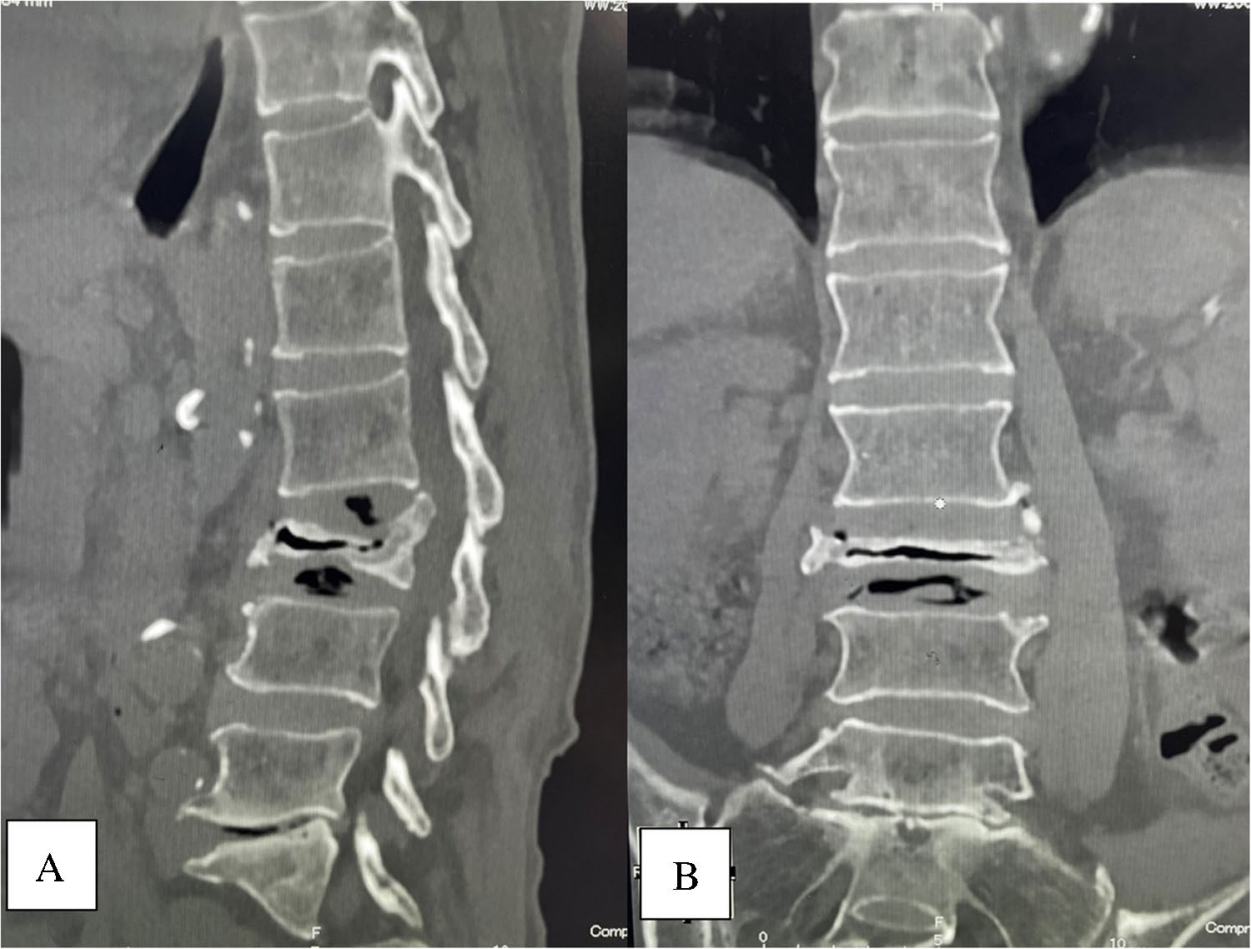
Intravertebral vacuum cleft was visible on sagittal (A) and coronal (B) views of computed tomography
